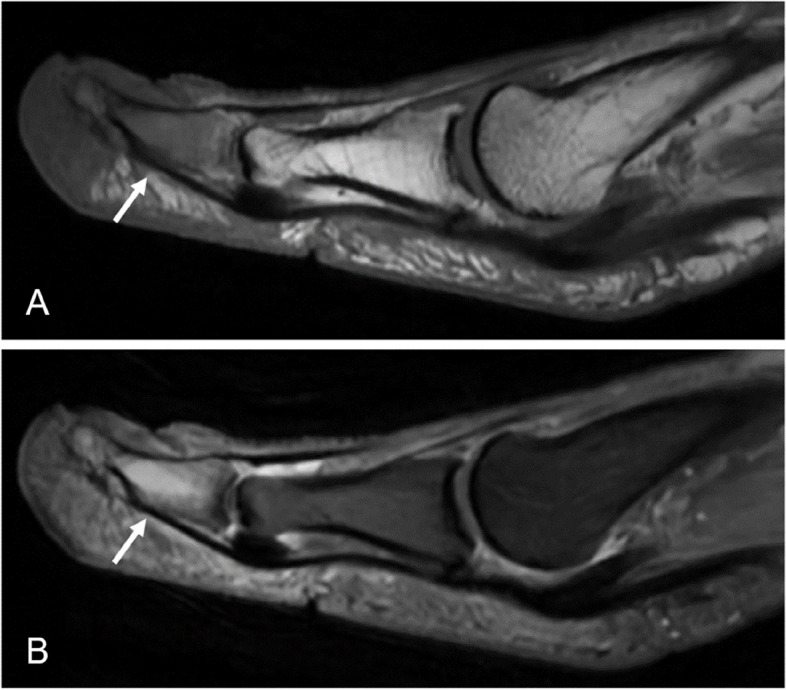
Initial magnetic resonance imaging of the phalanx of the first toe showing signs of osteomyelitis. In T1 weighted imaging loss of bone marrow signal in the distal phalanx of the digitus I can be seen (White arrow, Image A). In synopsis with hyperintense presentation of the affected segment in proton-density weighted imaging (White arrow, Image B) confirming bone edema, focal osteomyelitis was suspected
